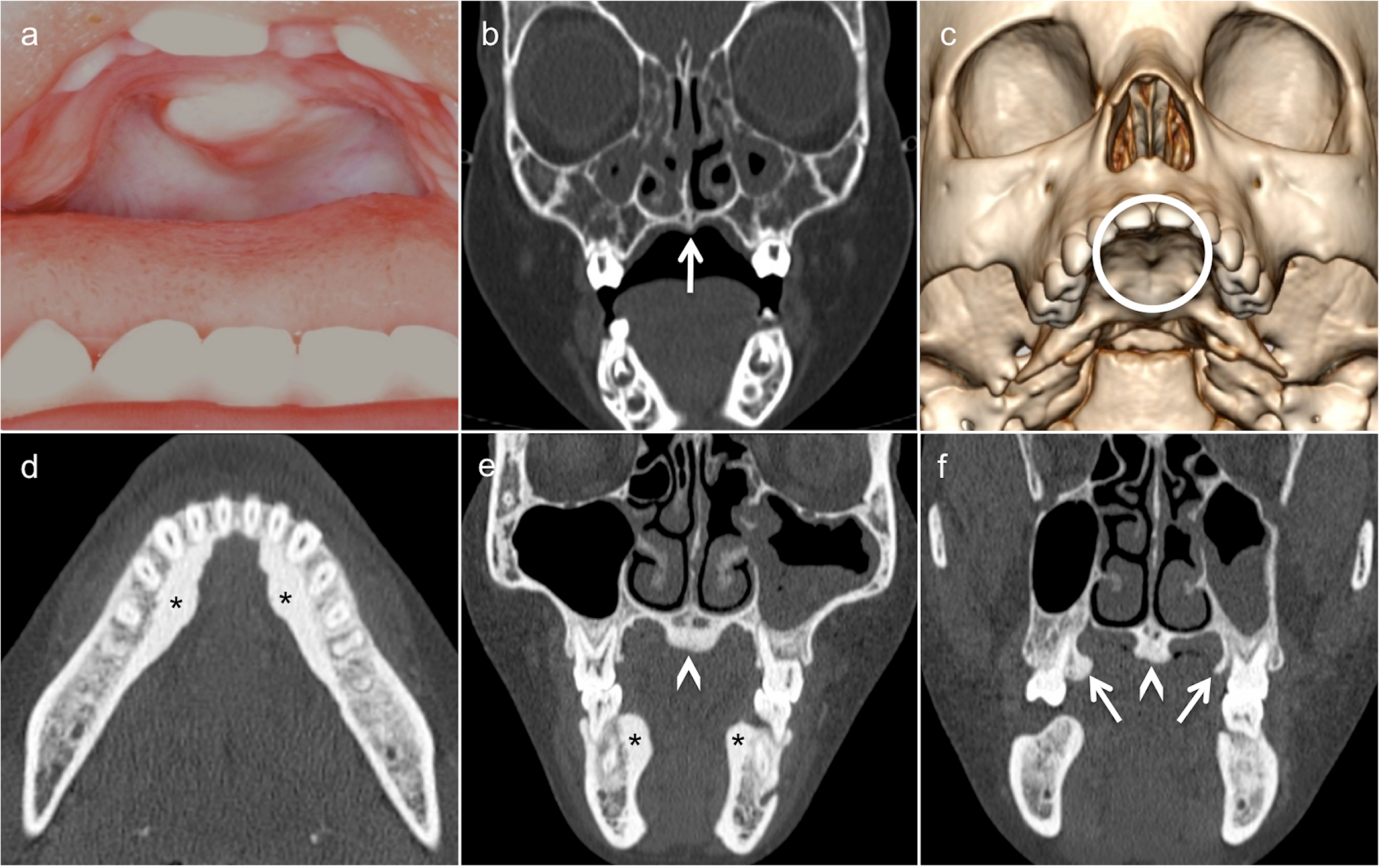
Torus palatinus (TP) in a 3-year-old girl who underwent imaging because of an indurated palpable midline mass of the hard palate increasing in size (a) after a fall occurring from a swing hanging on a tree two weeks earlier. Coronal low-dose CT image (bone window) (b) and three-dimensional CT VR reconstruction show a small midline spur/exostosis (*arrow* and *circle*). Characteristic aspect of a torus mandibularis (TM) (*asterisks* in d and e), of a TP (*arrowheads* in e and f) and of a torus maxillaris (TMax) (*arrows* in f) as seen in a young adult. It is worthwhile mentioning that TM and TMax are extremely rarely diagnosed in children
